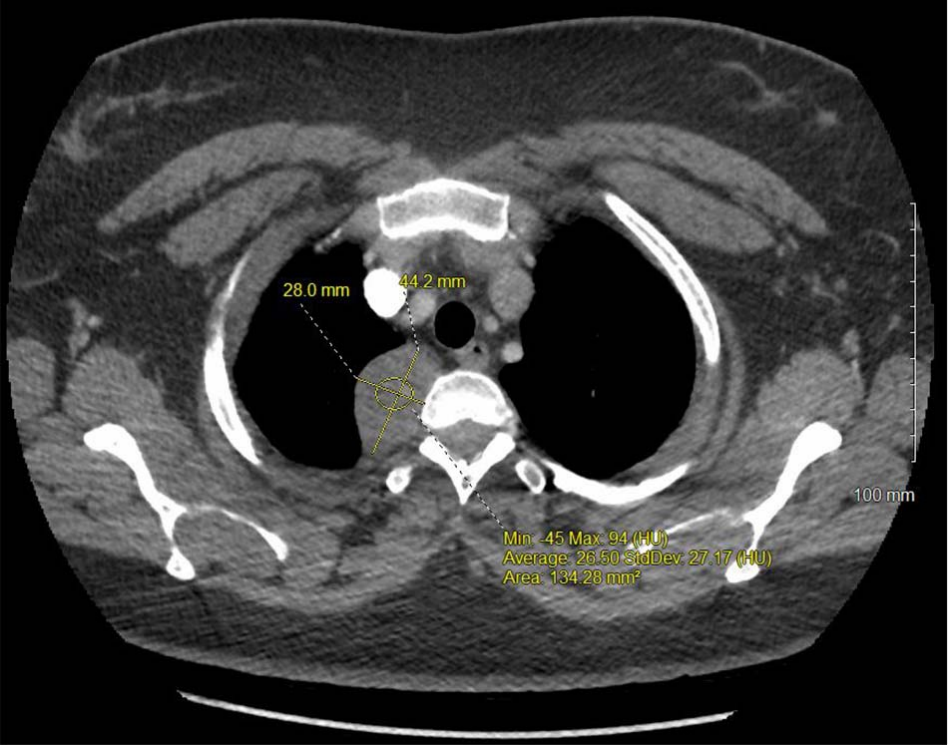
CT chest with IV contrast transverse view with detailed measurements of right T2-T3 paravertebral mass.
